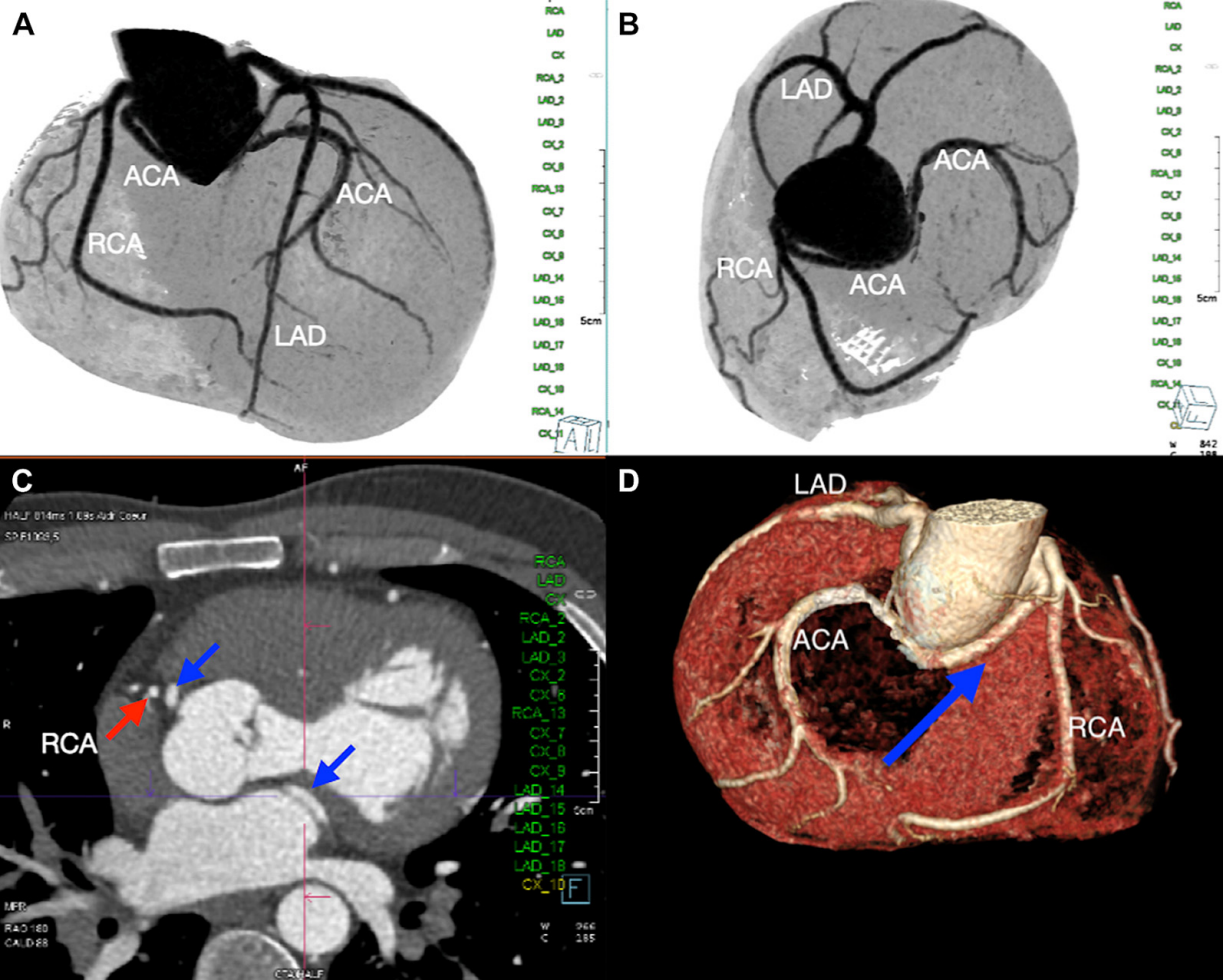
Computed Tomography of the ACA Computed tomography showing the pathway of the ACA (A, B) originating from the right coronary sinus and passing laterally along the non-coronary sinus. (C) The origin of the ACA is alongside the ostium of the right coronary artery (red arrow). (D) 3 D reconstruction of the pathway of the aberrant circumflex artery (blue arrow). ACA = aberrant circumflex artery (blue arrow) arising from the right coronary ostium; LAD = left anterior descending coronary artery; RCA = right coronary artery (red arrows).
